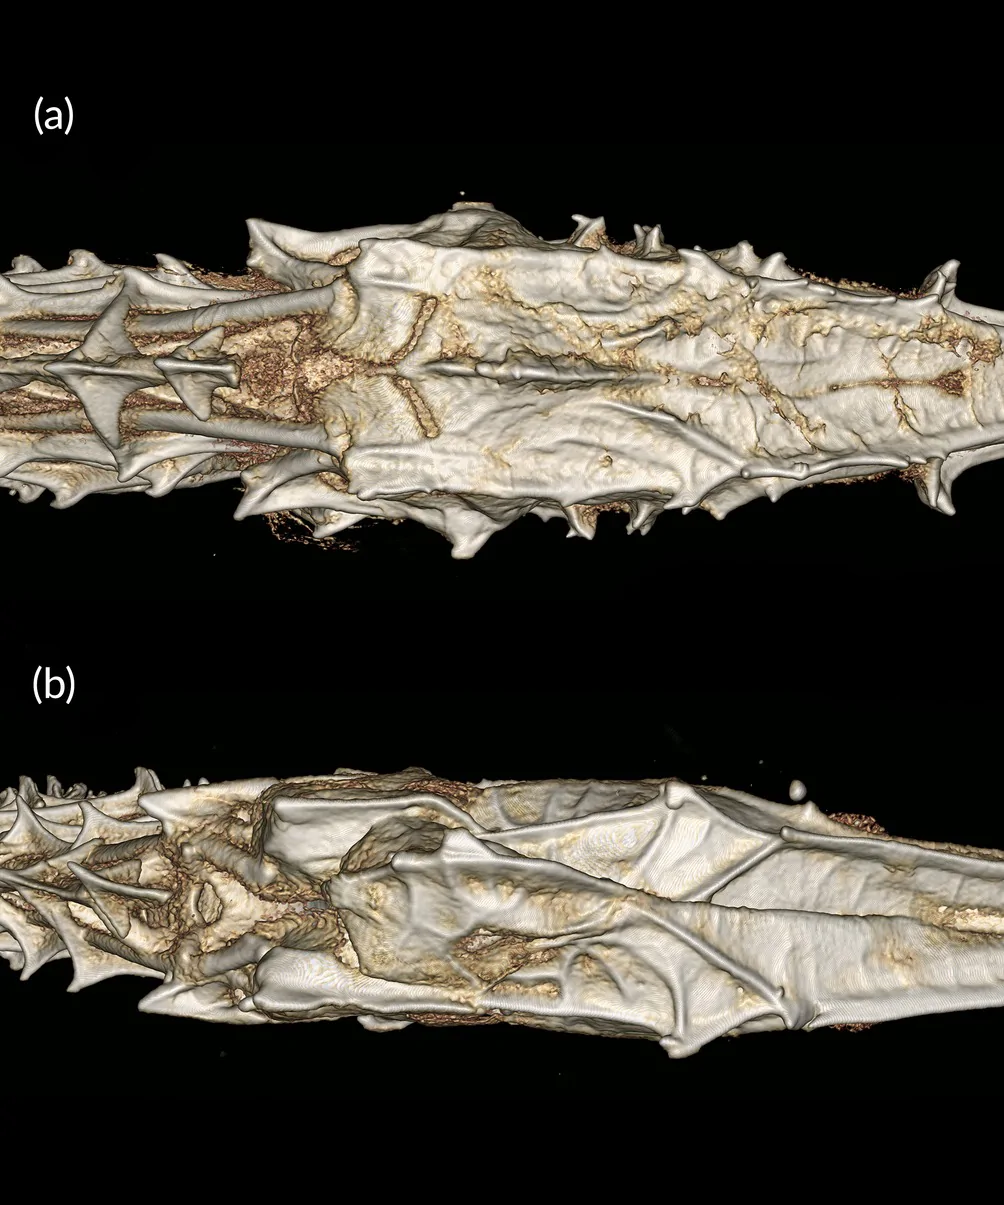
Micro‐CT reconstructions (dorsal view) of the supraoccipital crest of *S. snuffleupagus*: (a) holotype, female, AMS I.33751‐047; (b) paratype, male, NTM S.13600‐047.
